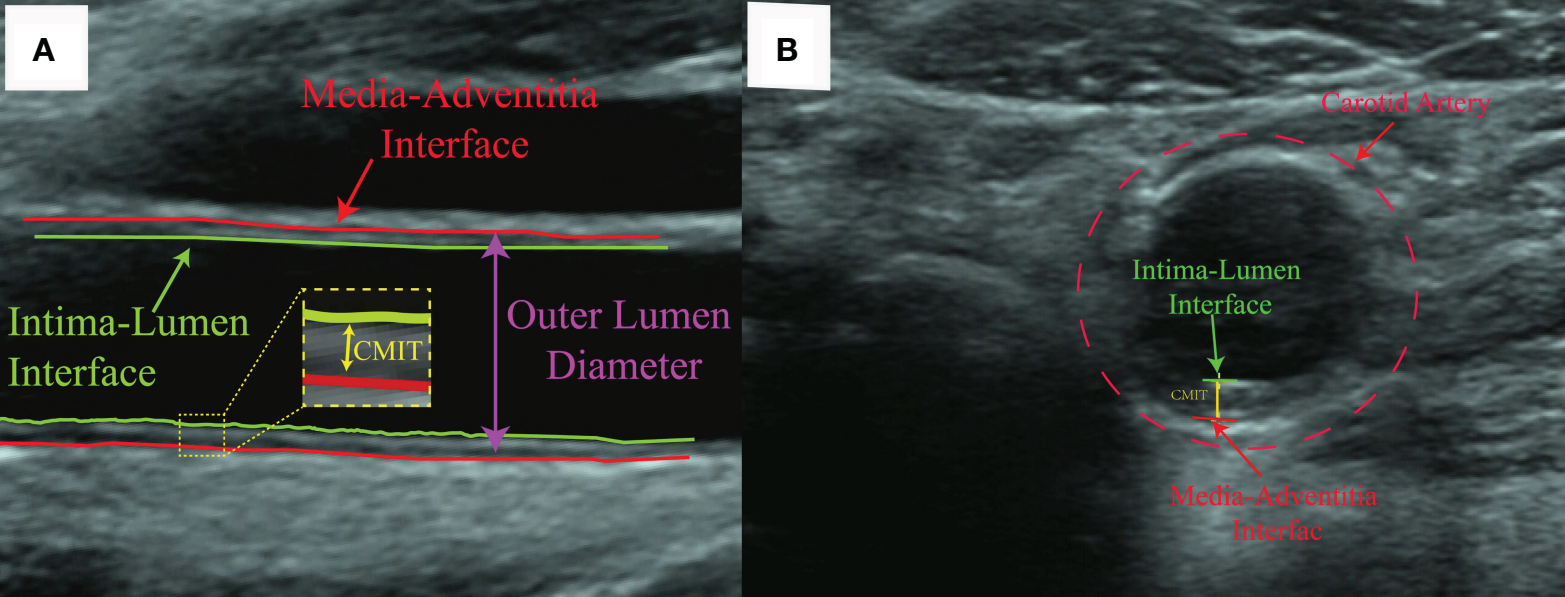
Ultrasound images of the cross-sectional and longitudinal sections for measuring the thickness of CMIT. (A) is a longitudinal ultrasound view of the carotid artery, where the green line indicates the Intima-Lumen Interface and the red line marks the Media-Adventitia Interface, with the CIMT measured in the yellow dashed area. (B) is a cross-sectional ultrasound view of the carotid artery, with the CIMT located between the green and red dashed lines, enclosed by the red dashed circle indicating the artery’s boundary.
